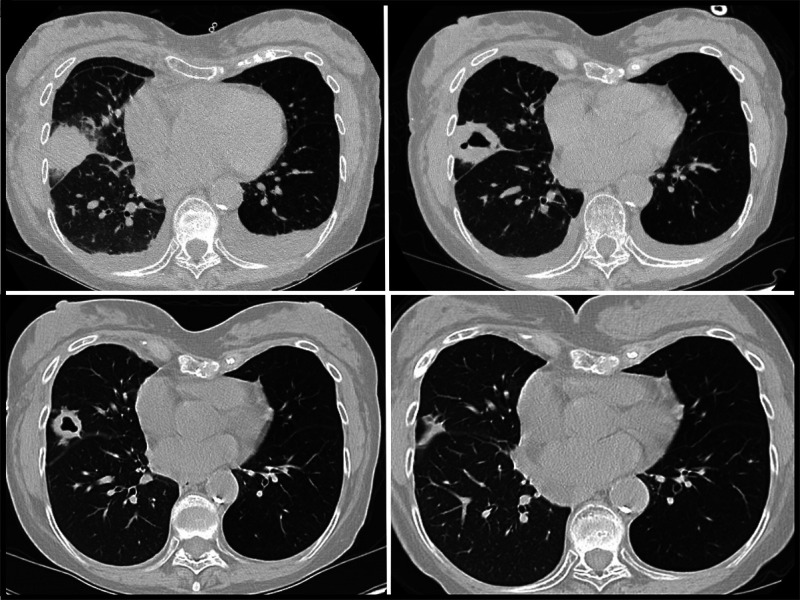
Computed tomography studies of the chest of patient A, who experienced infection with *Legionella* sp. (other than *Legionella*
